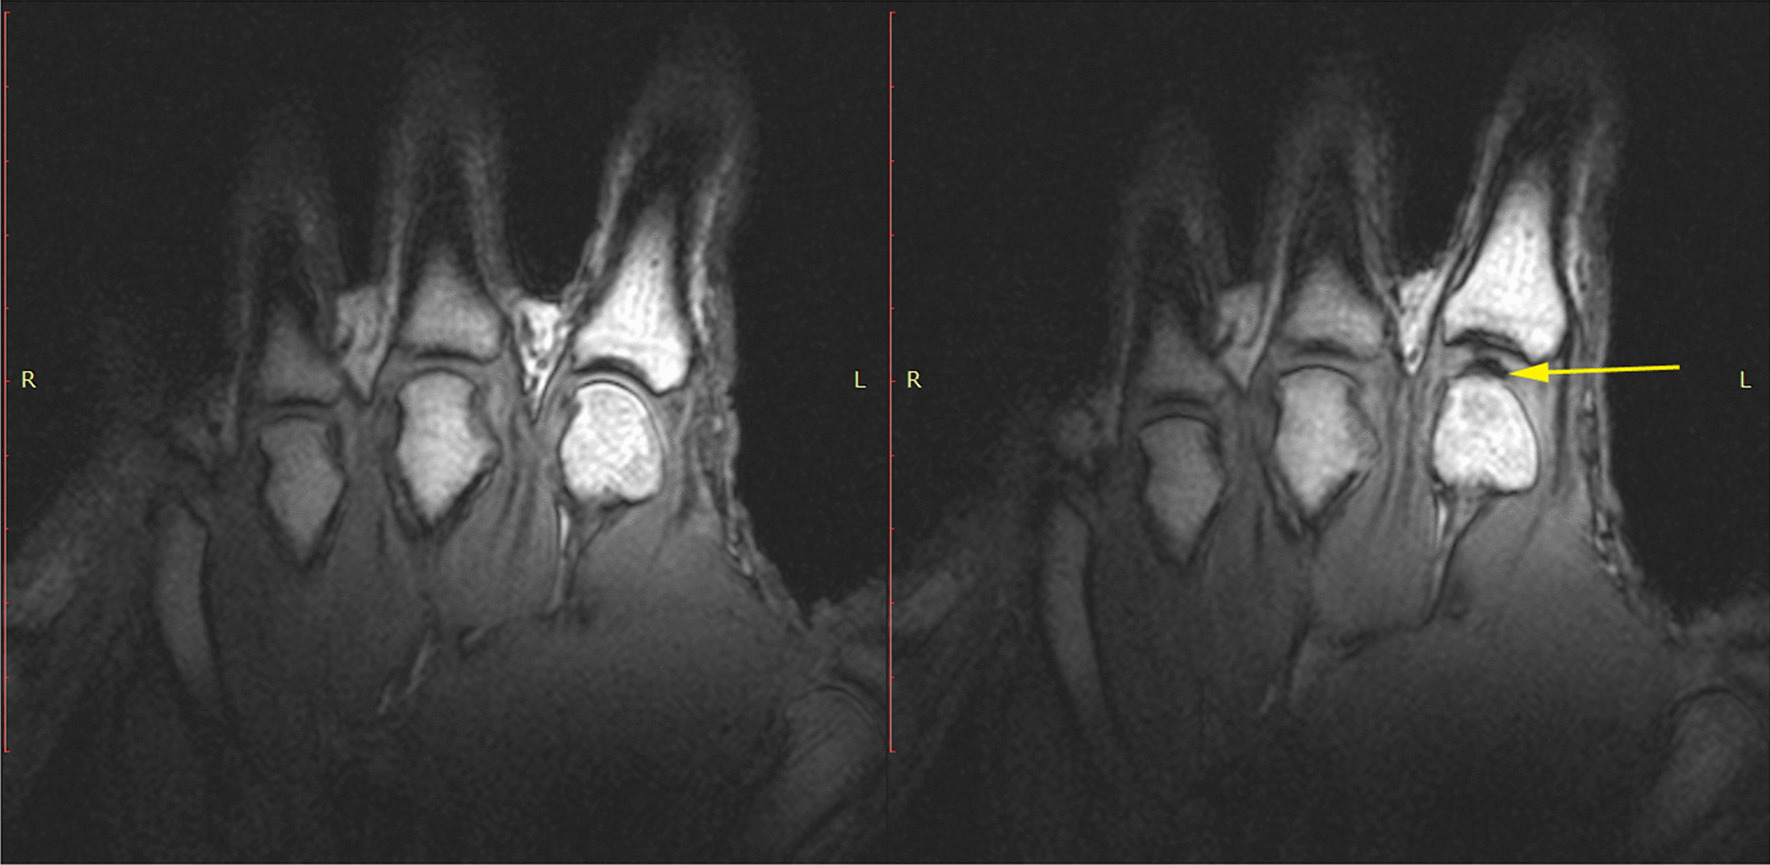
MR images before and after cavitation in an MCP joint. The original caption of this figure, reproduced from Herzog et al. [33], was “T1 static images of the left hand in the resting phase before cracking (left). The same hand following cracking with the addition of a post-cracking distraction force (right). Note the dark, intraarticular void (yellow arrow)”
